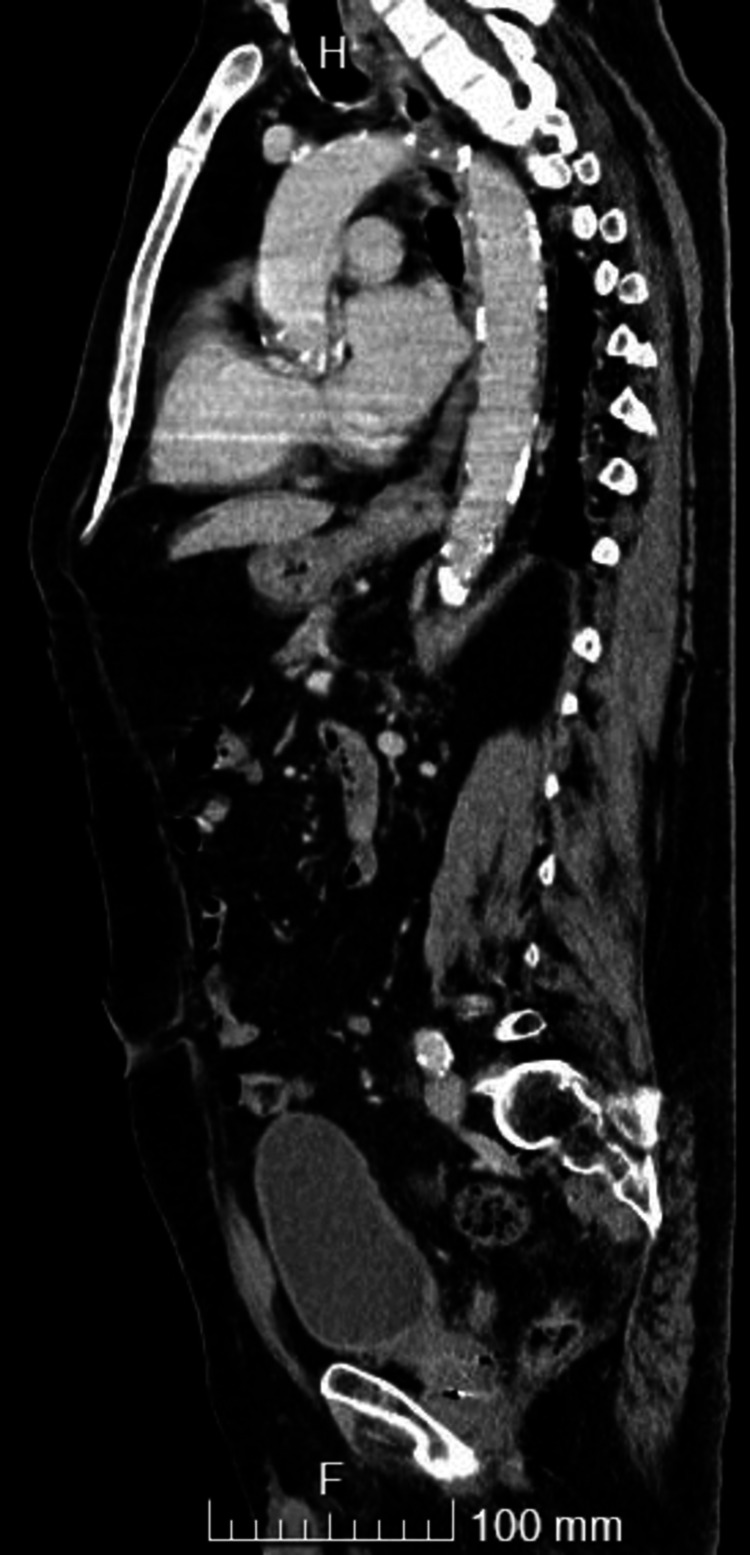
Thoracoabdominal-pelvic CT: calcified atheromatosis of the entire aorta.
